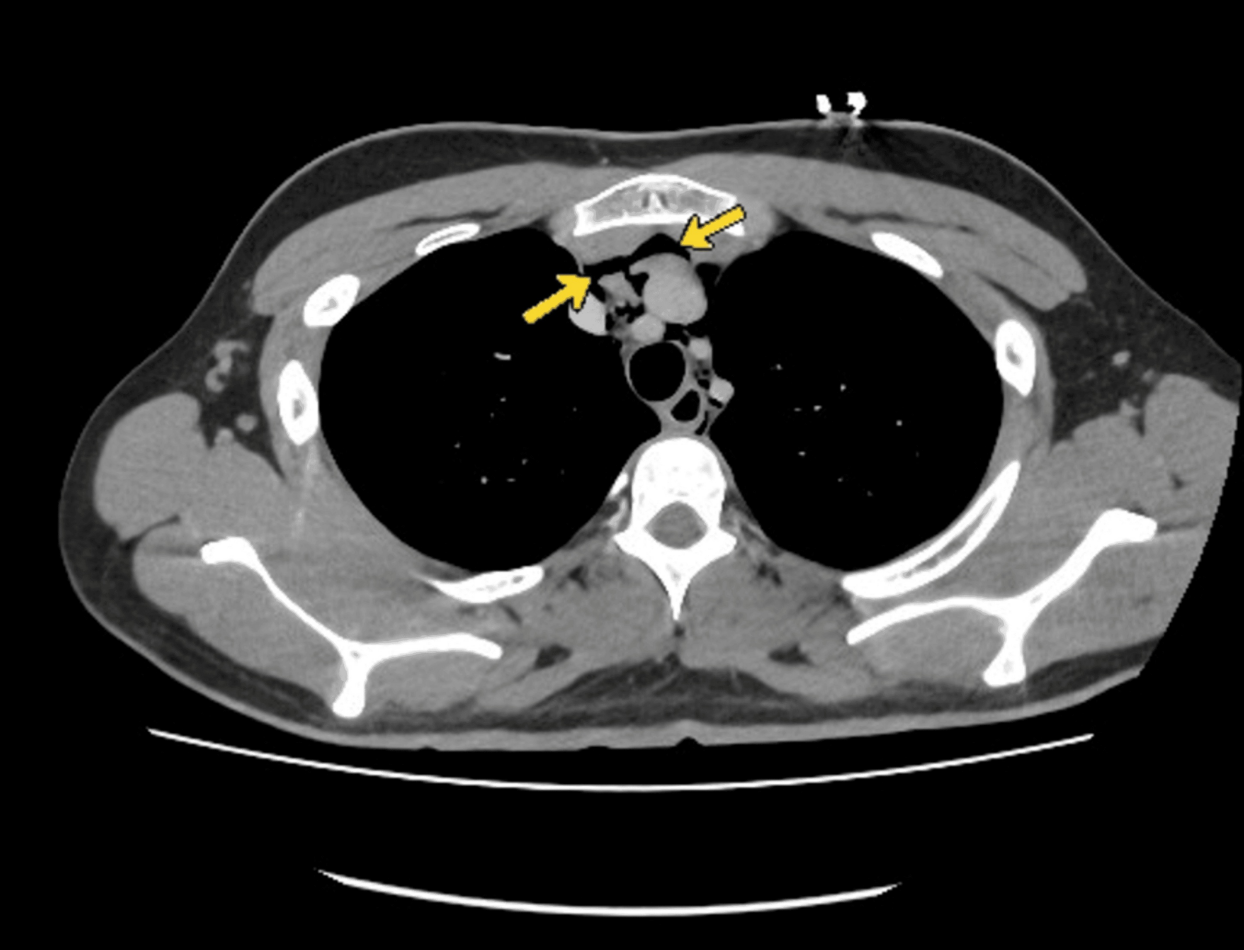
CT Chest with oral contrast showing pneumomediastinum
